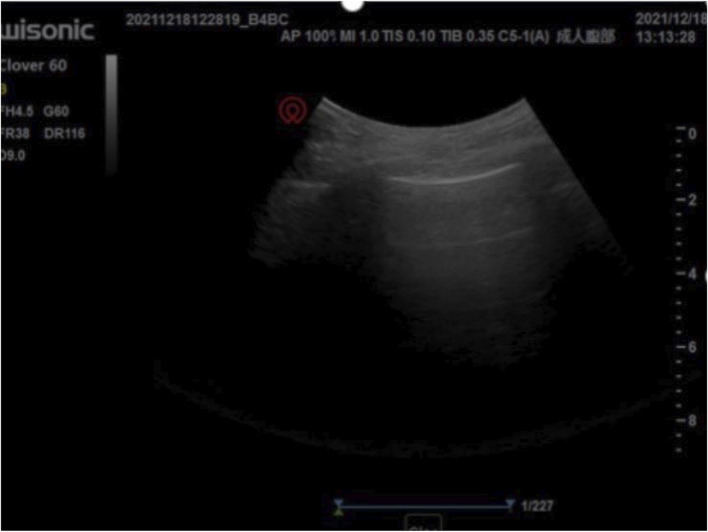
There were no obvious signs of increased extravascular lung water preoperatively. No B-line was found in preoperative and intraoperative lung ultrasonography, and only A-lines were found.
